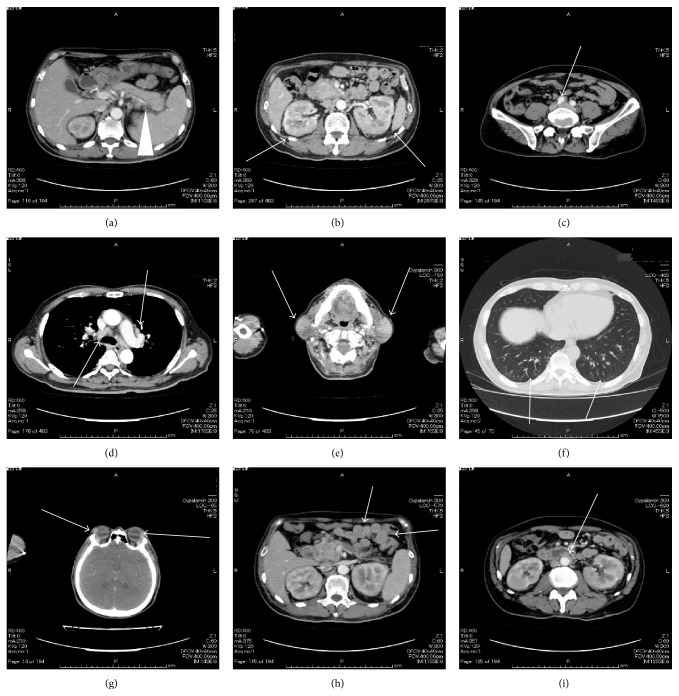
Contrast-enhanced computed tomography. It showed diffuse enlargement of the pancreas which involved something like a capsule that did not show enhancement in the early stage and showed enhancement in the late stage around the rim of the body and caudal portion ((a), white arrow), splenomegaly ((a), white arrowhead), multiple, small, low-attenuation lesions with swelling in bilateral kidneys ((b), white arrows), soft tissue shadow around the right common iliac artery ((c), white arrow), swelling of mediastinal and pulmonary hilar lymph nodes ((d), white arrows), swelling of bilateral submandibular parotid glands ((e), white arrows), centrilobular shadows in both lower lobes ((f), white arrows), swelling of the lacrimal glands ((g), white arrows), edematous changes of jejunum ((h), white arrows), and soft tissue around the abdominal aorta ((i), white arrow).
